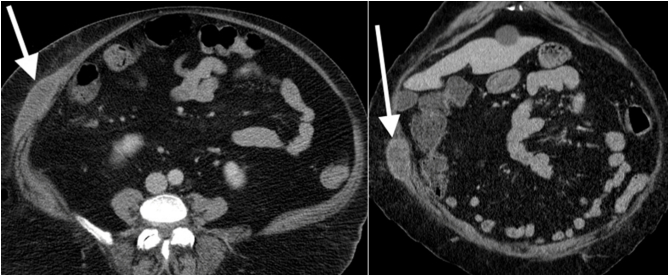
LEFT: Axial contrast-enhanced CT image shows an oblong lesion within the soft tissues of the abdominal wall. RIGHT: Coronal CT image of the same lesion.
